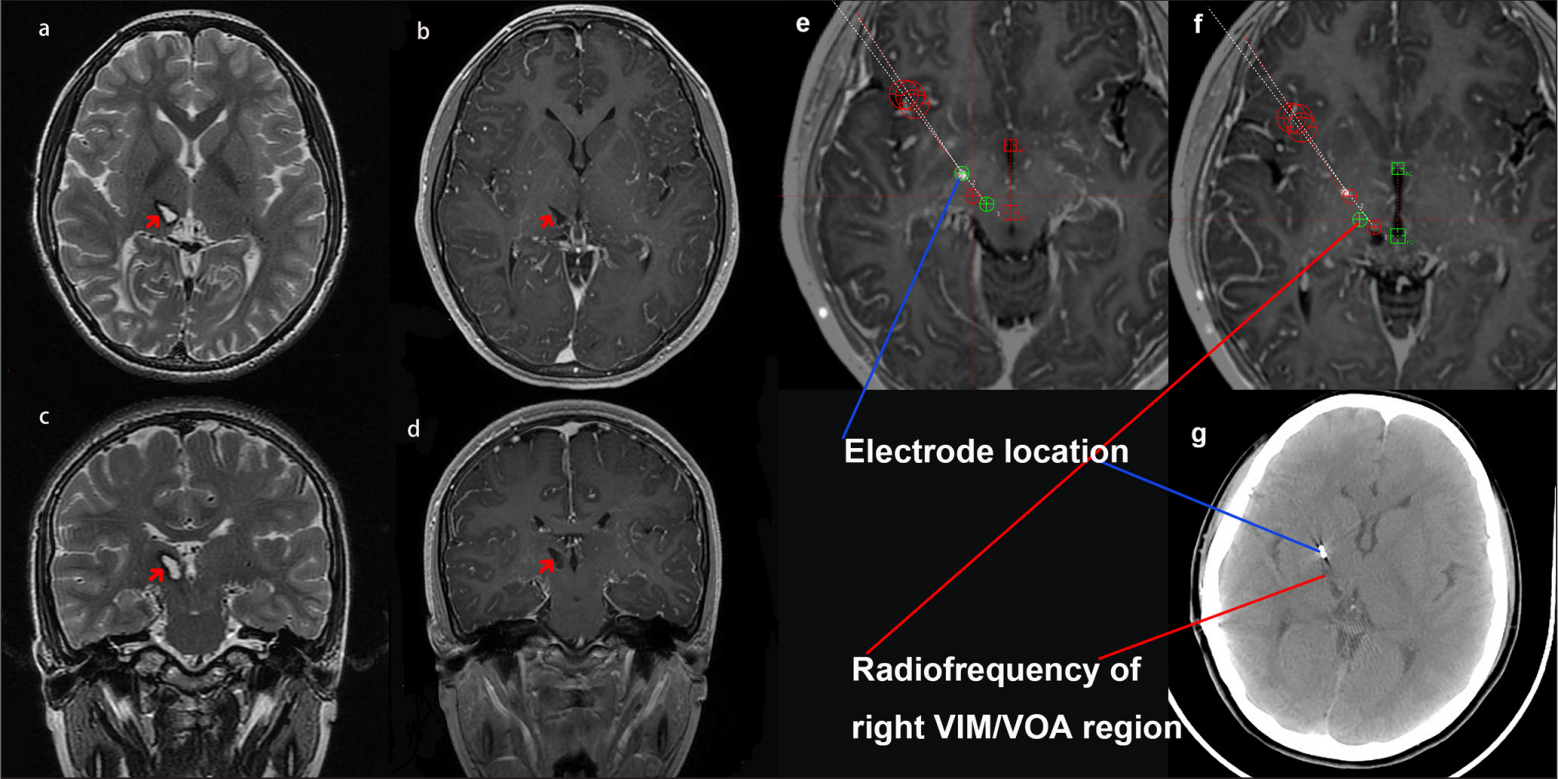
a–d (a and b: axial view; c and d: coronal view): Brain magnetic resonance imaging before stereotactic surgery revealing abnormal signal intensities in the right thalamus (red arrows). e–g: Stereotactic targeting images. Electrode location is marked with a blue line, and ventral intermediate nucleus/ventral oral nucleus radiofrequency lesion is marked with a red line.
